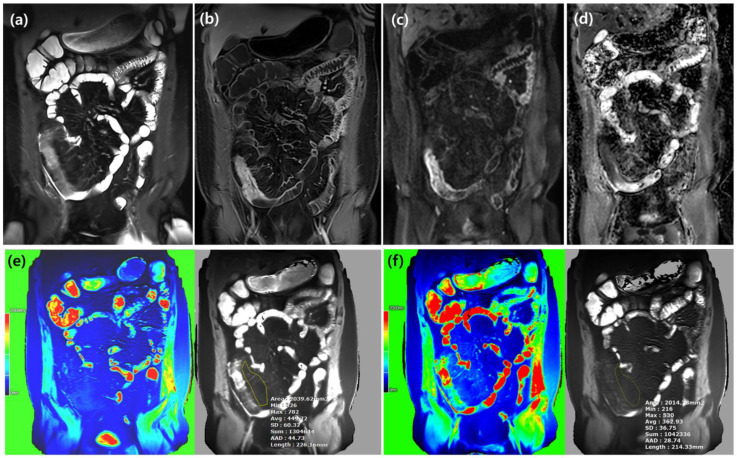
Images from a patient with moderately active inflammatory Crohn’s disease (CDAI = 385.1). The thickened terminal ileum exhibits mural and perienteric edema (a), mural enhancement, and prominent vasa recta (b). Restricted diffusion is seen on DWI b-900 (c) and ADC map (d). The coronal native T1 map (e) demonstrates a T1 relaxation time of 449.7 ms, exceeding the median native T1 value reported for active disease patients. Post-enhanced T1 map (f) demonstrates a T1 relaxation time of 362.9ms. ΔT1 was 86.8 ms.
